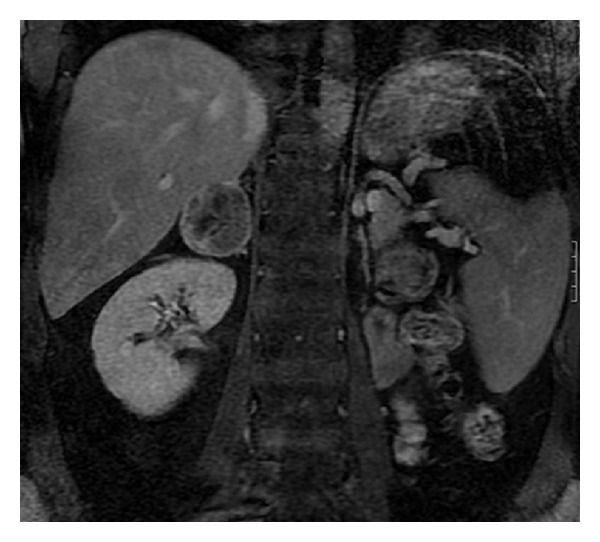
Preoperative magnetic resonance imaging (MRI) of right peri-adrenal tumor.
